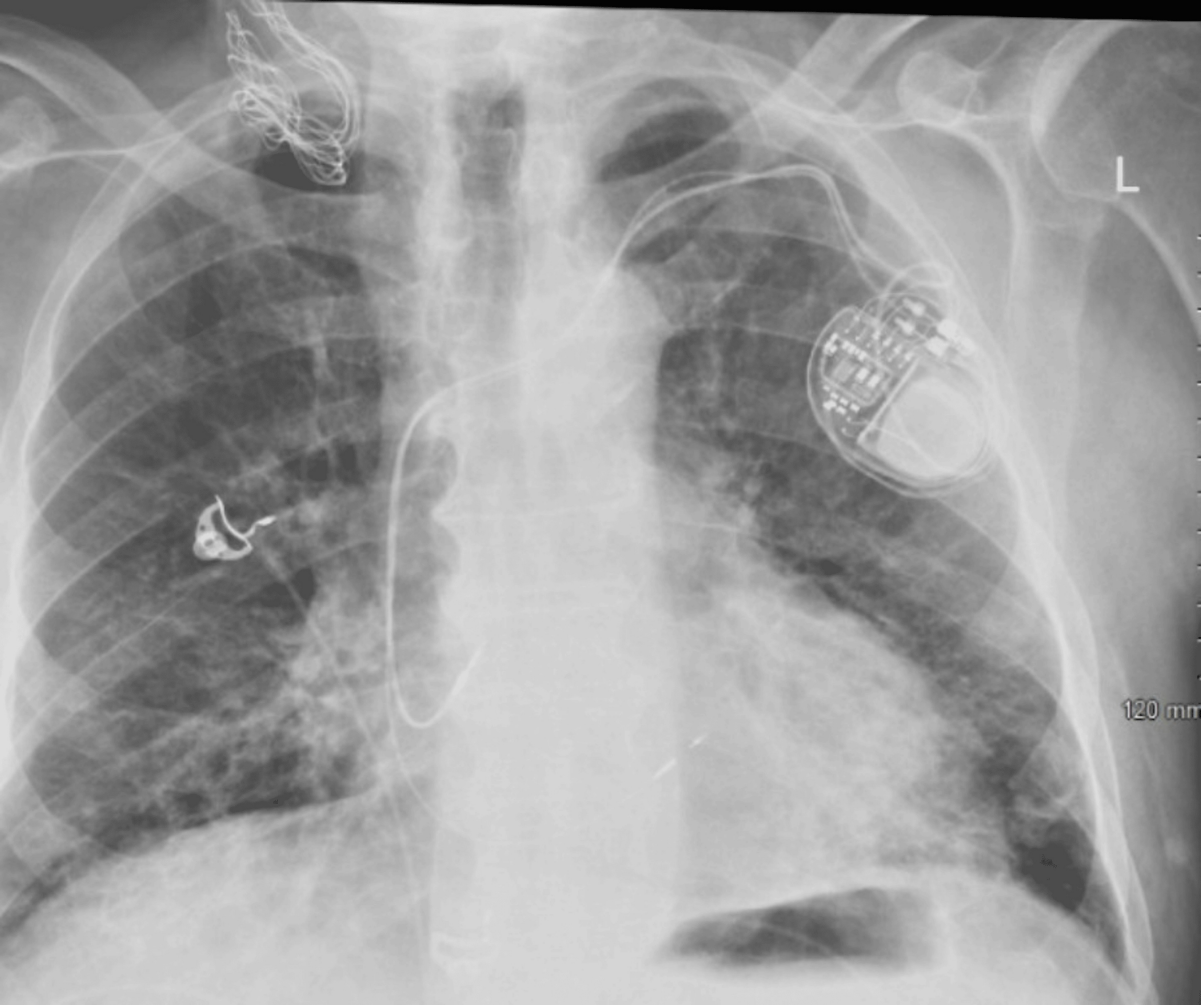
Chest X-ray showing dual-chamber pacemaker placement
